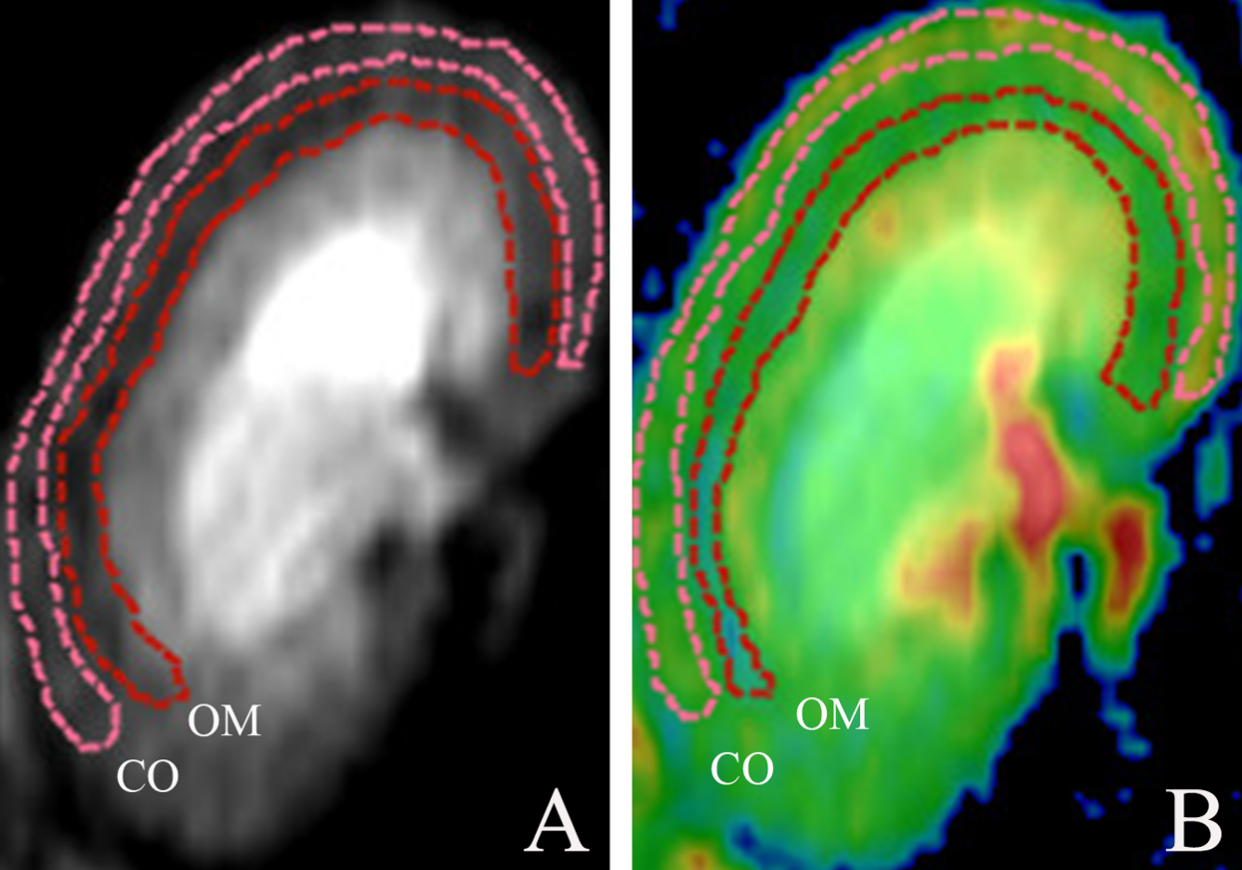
Morphologic MR images with representative cortical and medullary ROI segmentations. (A) ROIs traced on DTI of kidney and (B) ROIs traced on DTI pseudocolor map. DTI, diffusion tensor image; ROI, region of interest; CO, cortex; OM, outer medulla.
